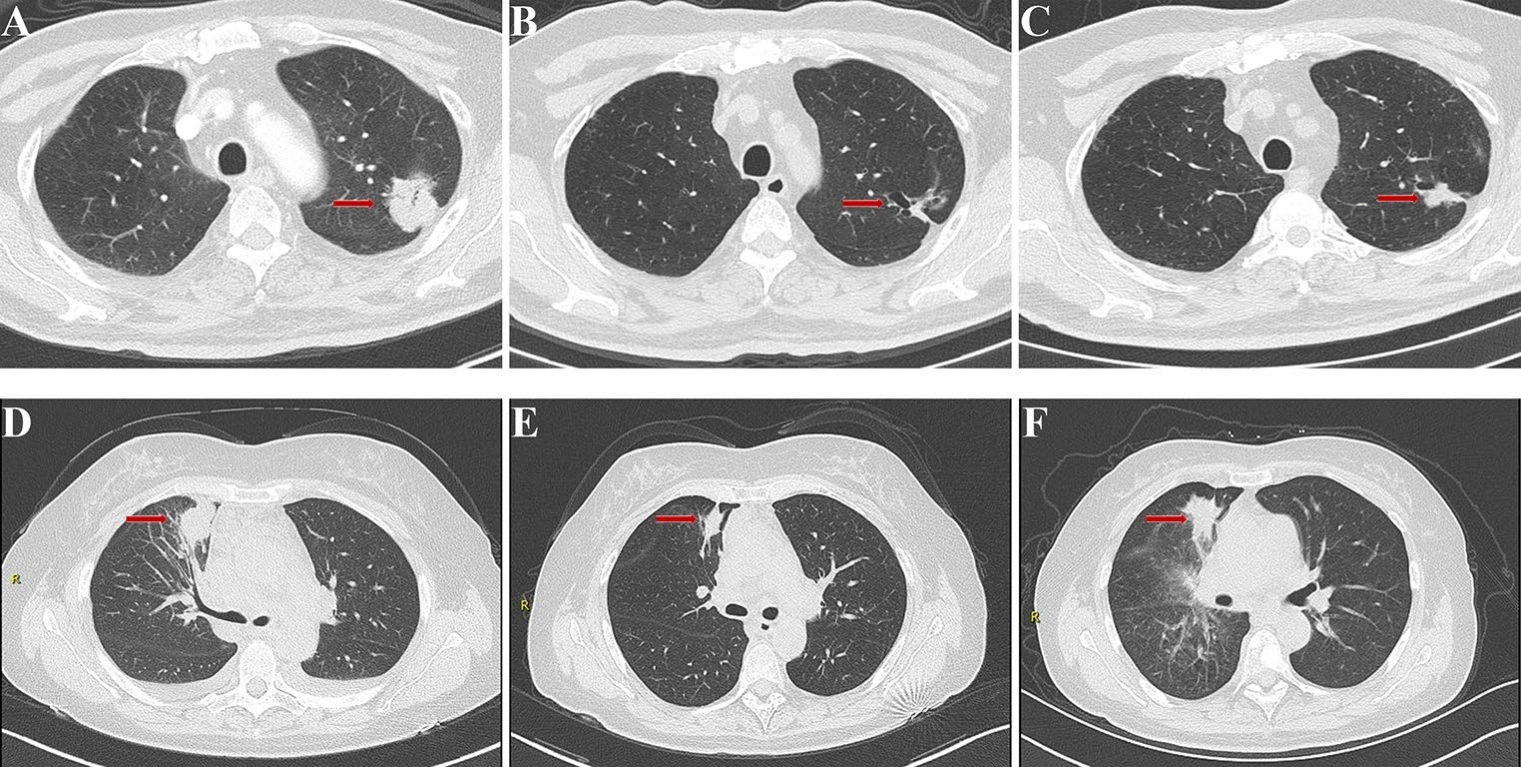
Example of CT images from pre-treatment to progression of two patients with different ABC-Scores. CT images before icotinib treatment (A), at the time of partial response (B), and at the time of disease progression (C) of a 60-year-old woman with the ABC-Score equal to 1. CT images before icotinib treatment (D), at the time of partial response (E), and at the time of disease progression (F) of a 57-year-old woman with the ABC-Score equal to 3
